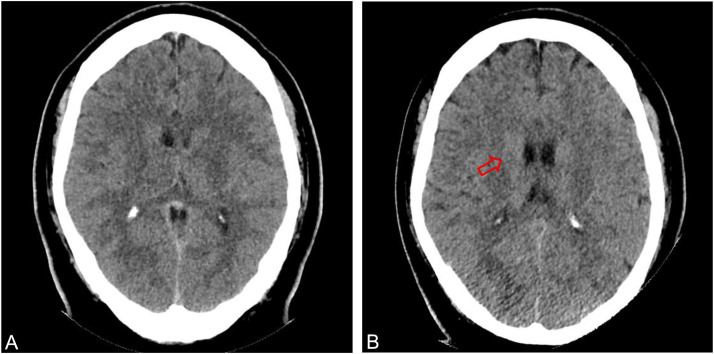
Axial Initial noncontrast CT brain (A) Two days after presentation axial postcontrast CT brain (B)- showing an enlarged right. Caudate head and ill-defined hypodensities in the right caudate and thalamus. Note that lack of contrast enhancement on the CT performed 2 days after presentation.
